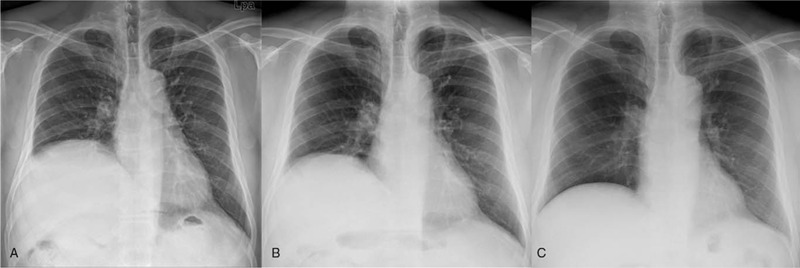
(A) Right hemidiaphragmatic elevation on the initial chest radiograph. (B) No interval change at postoperative 1 month. (C) Remarkable descent of the right diaphragm 3 months after surgery.
